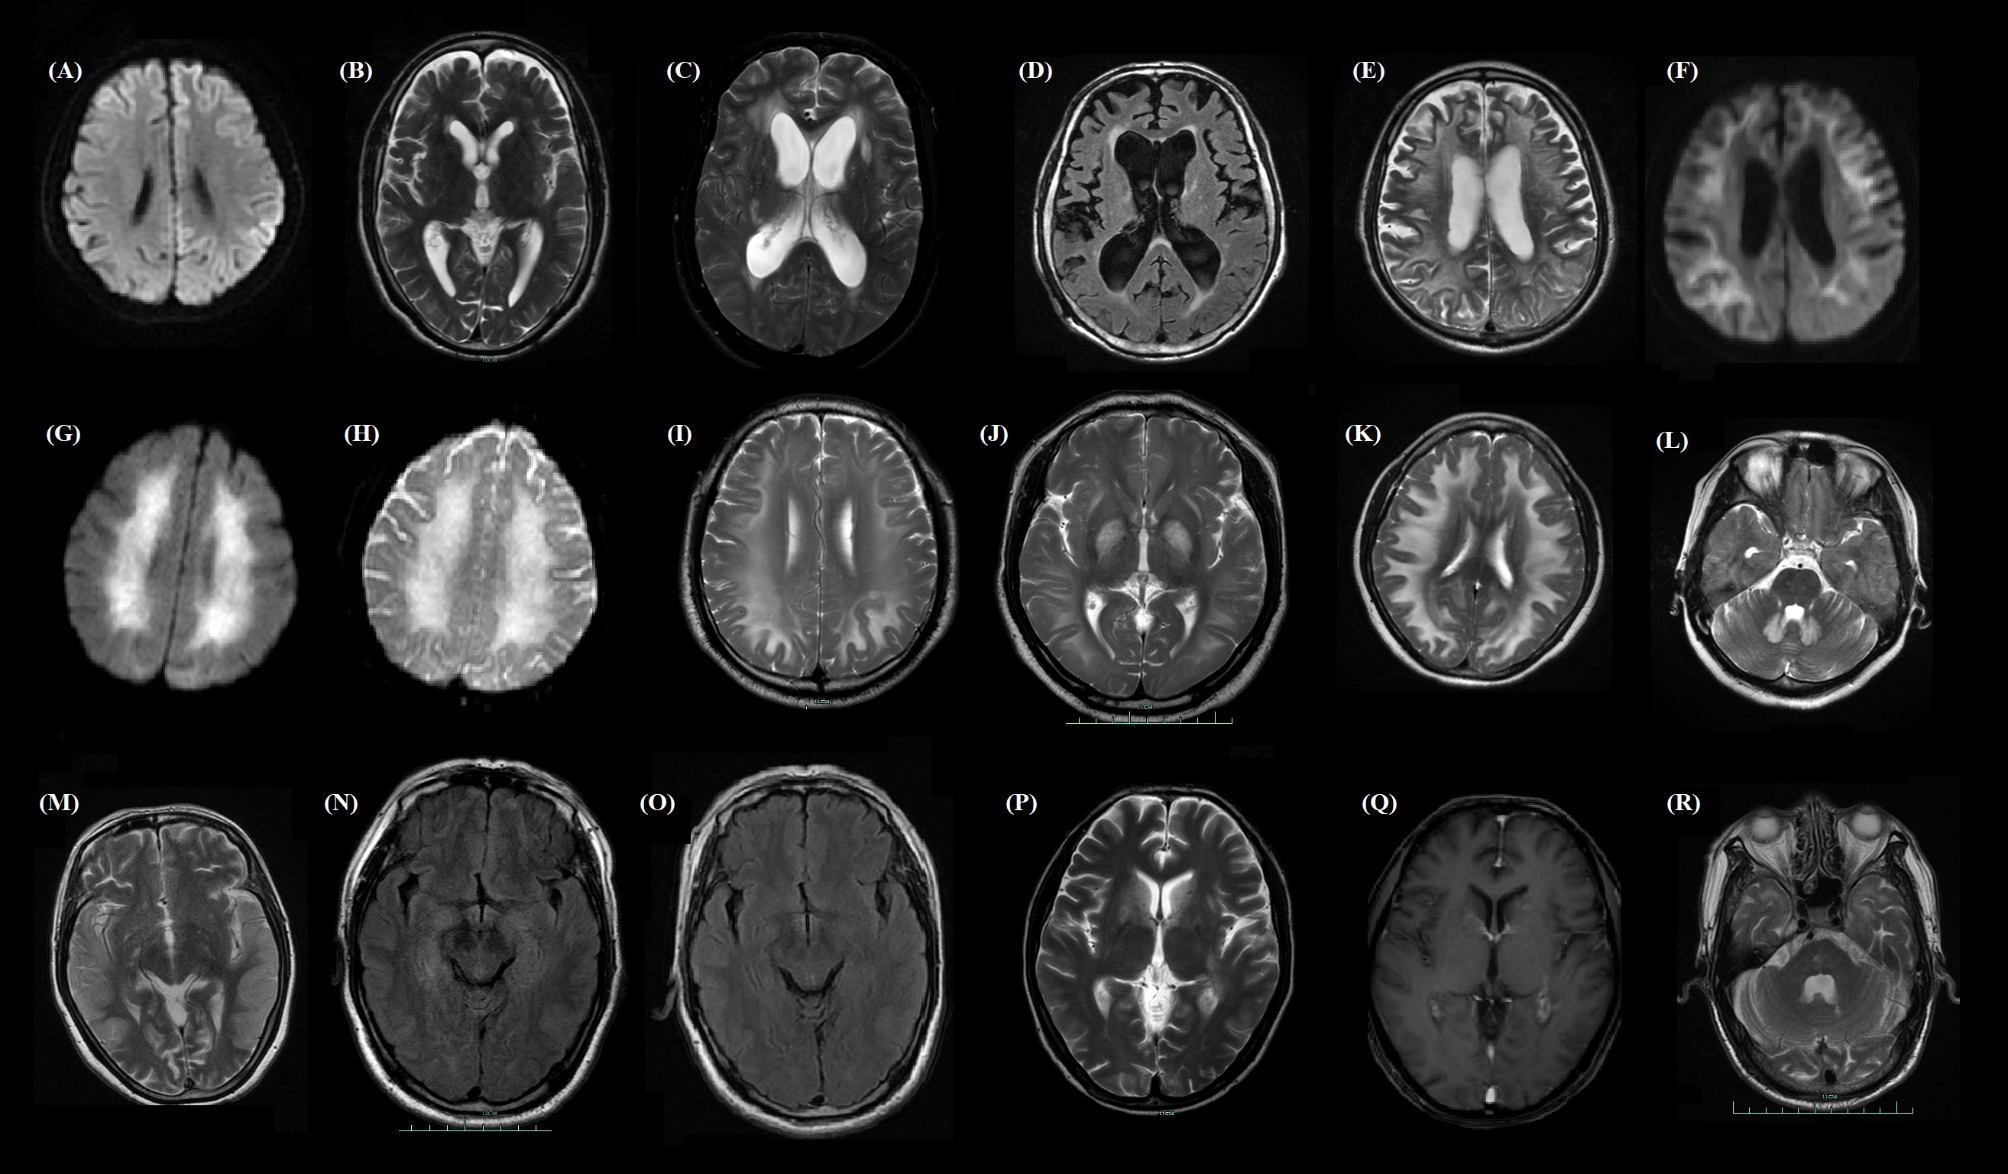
Selected brain MRI images in participants with rapidly progressive dementia. The descriptive text of each image is shown with definitions of disease name, age, sex, disease course, and MRI data. (A) Creutzfeldt–Jakob disease: 72 years, female, 20 days. DWI image indicating the bilateral frontal and parietal cortex hyperintensities. (B) Neurosyphilis: 61 years, male, 2 months. T2WI demonstrating wide brain atrophy. (C) Tuberculous meningoencephalitis: 55 years, male, 1 month, T2WI demonstrating ventricular enlargement, paraventricular hyperintensities and increased vascular shadow. (D) Alzheimer’s disease: 75 years, male, 1 year, T2WI demonstrating wide brain atrophy and ventricular enlargement. (E & F) Neuronal intranuclear inclusion disease: 78 years, male, 2 months, T2WI demonstrating diffuse white matter hyperintensities and brain atrophy, and DWI images indicating hyperintensities involving corticomedullary junction in the frontal and parietal lobes. (G & H): CO poisoning: 61 years, female, 1 month, DWI and ADC images indicating diffuse sub-cortical hyperintensities. (I & J) Polyvinyl alcohol poisoning: 35 years, male, 1 month, T2WI images showing diffuse subcortical and globus pallidus hyperintensities. (K & L) Dichloroethane poisoning: 34 years, female, 10 days, T2WI showing diffuse subcortical and dentate nucleus hyperintensities. (M) Mitochondrial encephalomyopathy with lactic acidosis and stroke-like episode: 52 years, female, half a month. T2WI showing hyperintensity and local swelling on the bilateral temporal lobes. (N & O) Autoimmune encephalitis associated with LGI1 receptor: 47 years, male, 20 days, FLAIR showing hyperintensities on the bilateral hippocampus (N), and lesions disappeared after treatment at 6-month follow-up (O). (P & Q) Sjogren’s syndrome with central nervous system involvement: 45 years, male, 2 months, T2WI showing bilateral basal ganglia (caudate nucleus, globus pallidus and anterior thalamus) symmetrical hyperintensities (P), and enhanced sequence showing significantly enhanced lesions in patches (Q). (R) Cerebral autosomal dominant arteriopathy with subcortical infarcts and leukoencephalopathy: 62 years, male, 2 years, T2WI images showing hyperintensity on the pole of the bilateral temporal lobes
